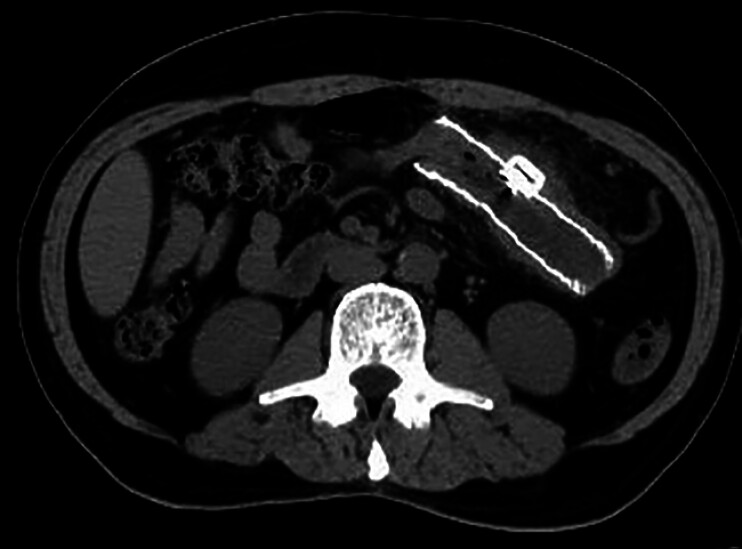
Computed tomography confirmed the placement of the stent plus vascular plug.
